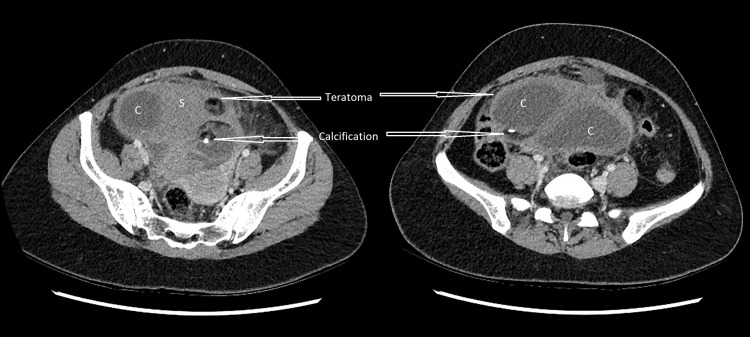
Axial images of CT abdomen Axial images of CT abdomen with intravenous contrast in the portal-venous phase. The two axial images show a teratoma with areas of fat, cystic (C) and solid (S) tissue and calcification. Surrounding fat stranding is visualised in both images.
